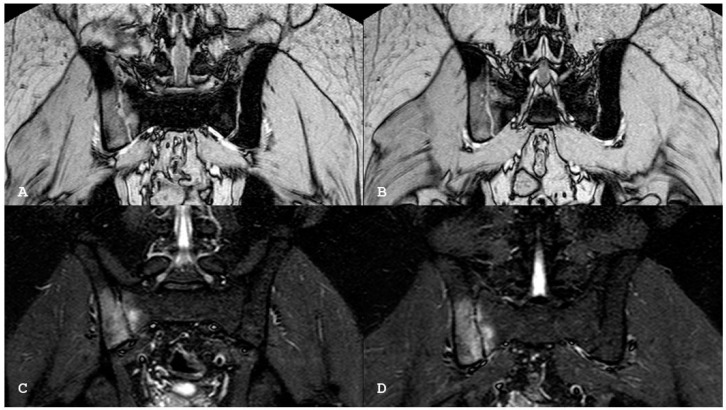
Oblique coronal gradient echo T2 sequences (A,B) and oblique coronal short tau inversion recovery (C,D) magnetic resonance imaging sequences show bony irregularity with subchondral erosions associated with the subchondral bone edema of both the sacral and iliac sides of the right sacroiliac joint.
